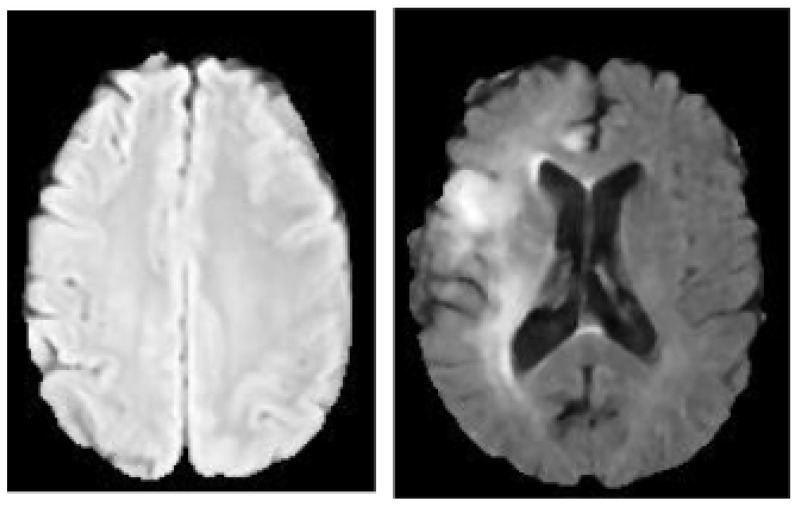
MRI brain images. The image on the left is Benign while the image on the right is Malignant.
